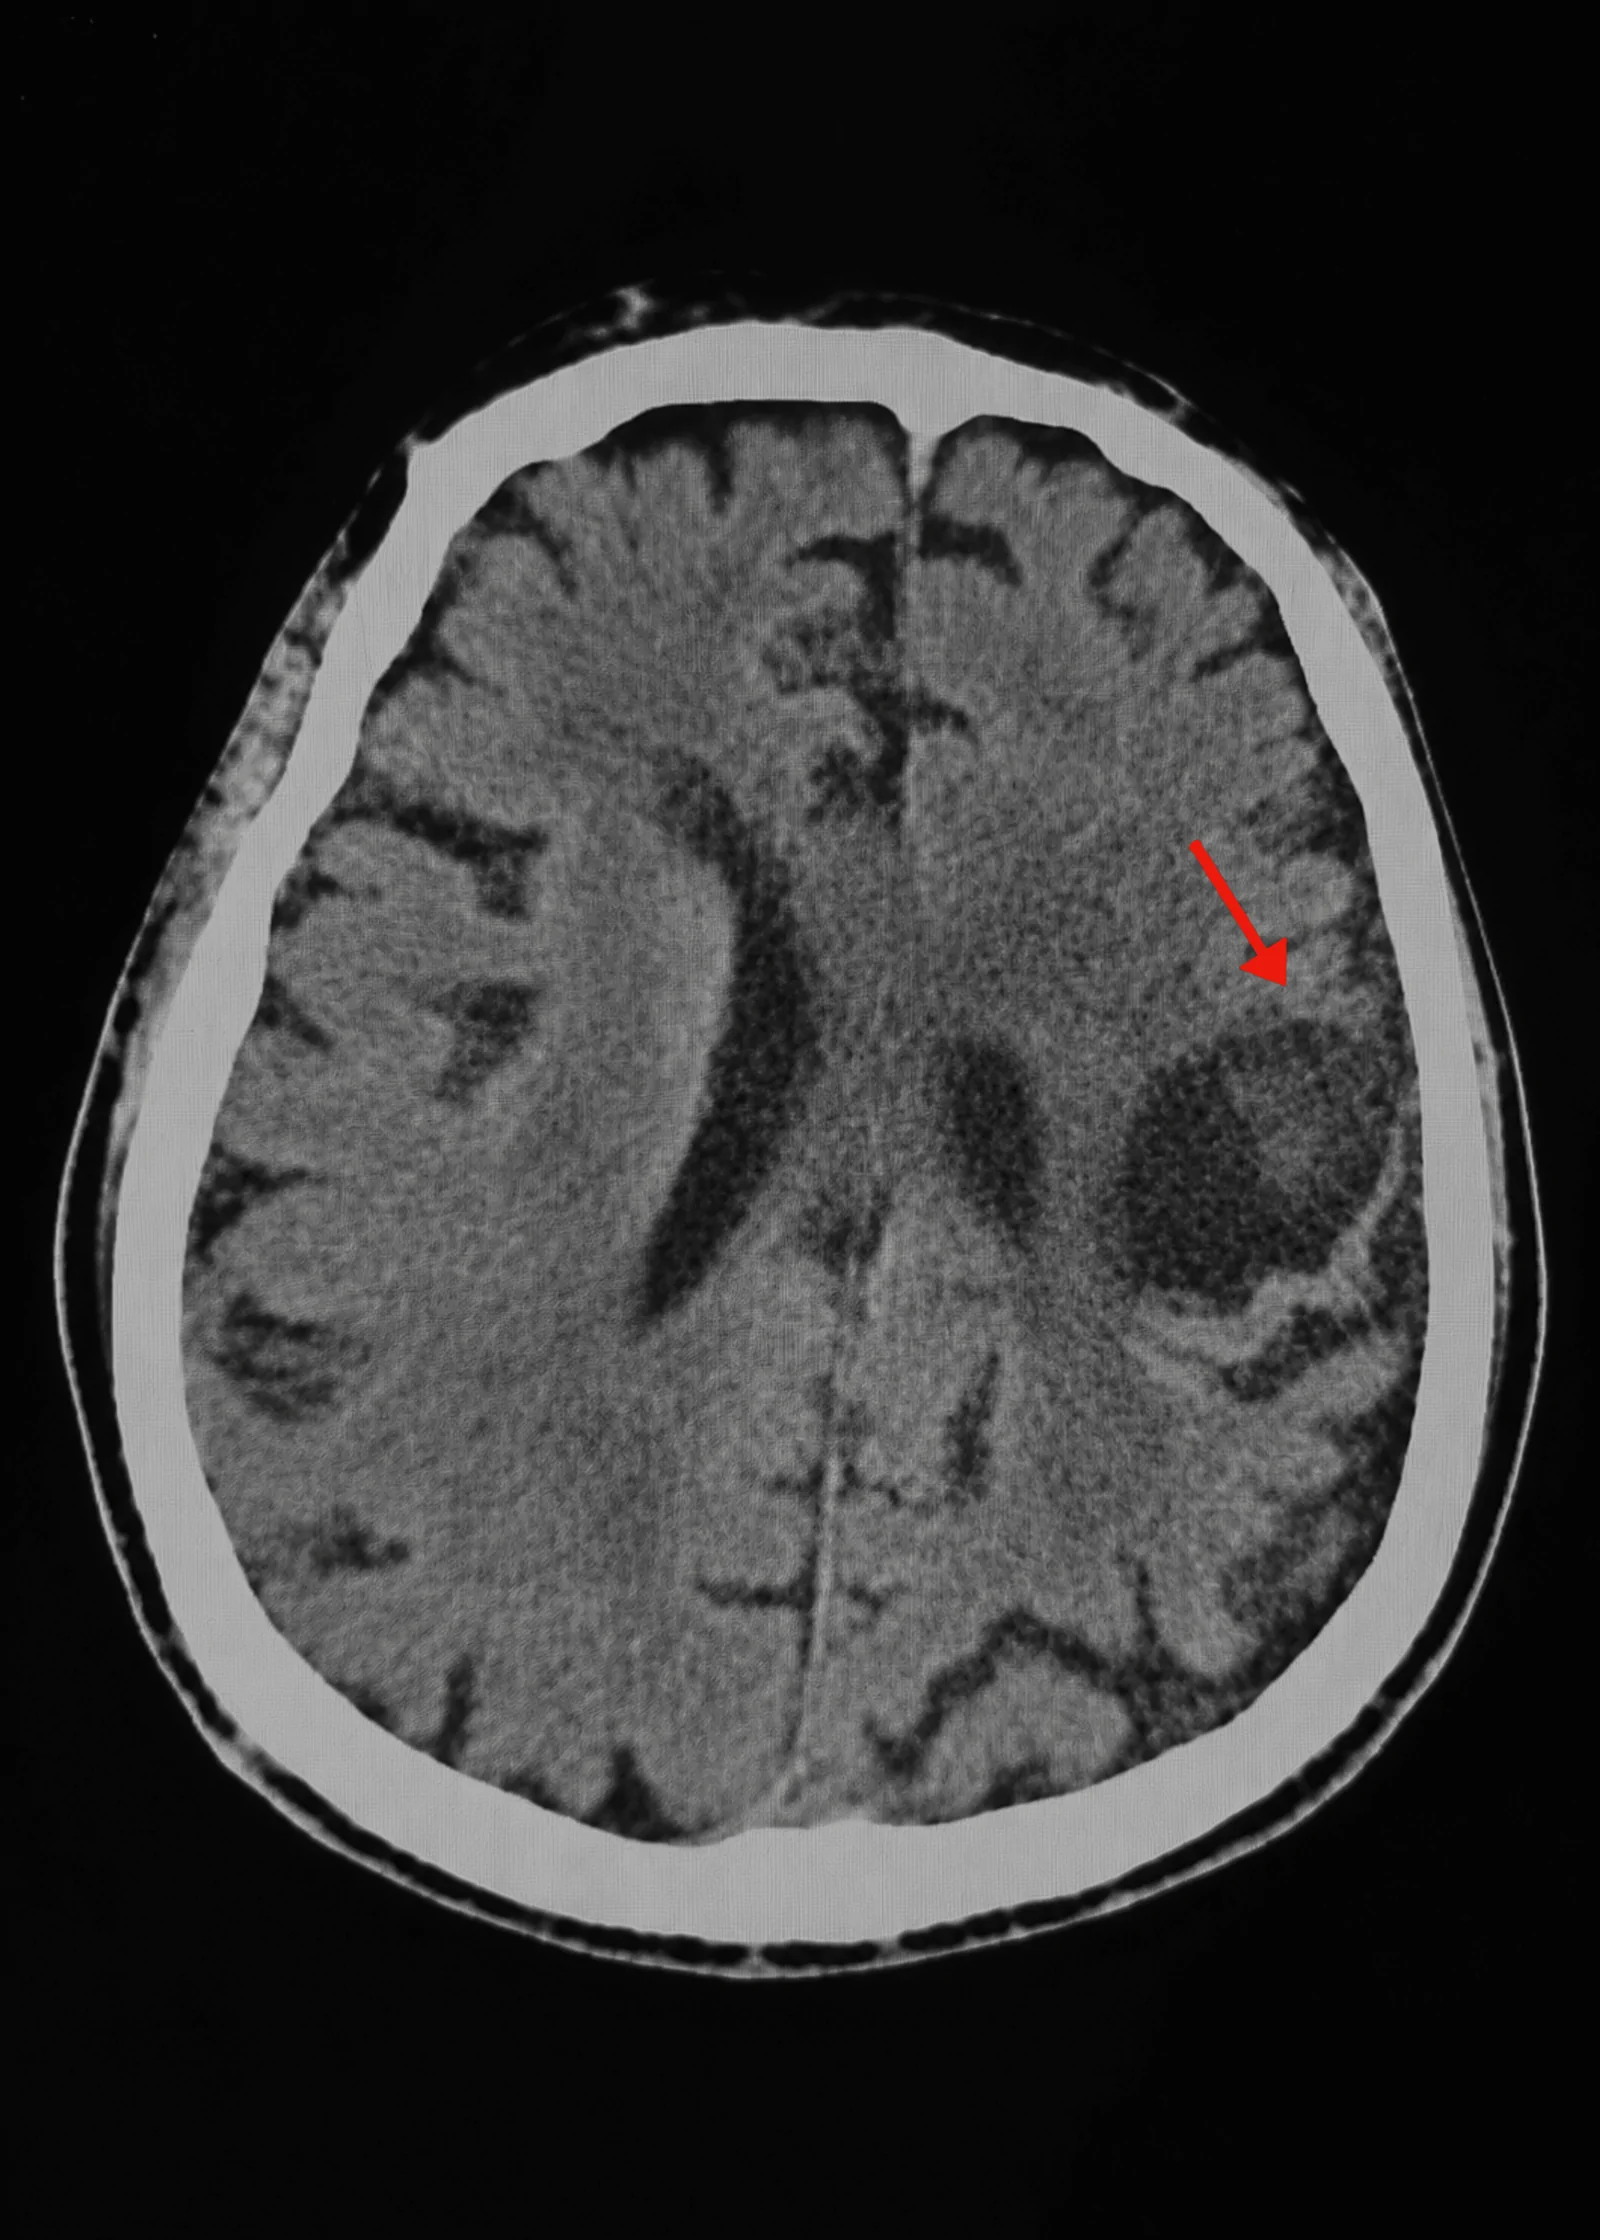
Non-contrast CT of the brain demonstrating a left frontoparietal chronic infarction involving the left middle cerebral artery territory, indicated by the red arrow.
